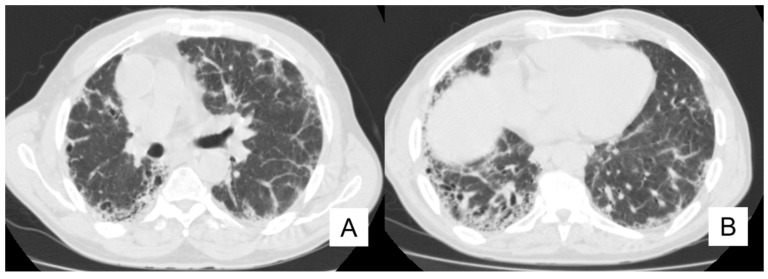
An 80-year-old man with values of SpO_2_, Forced Vital Capacity (FVC) and Forced Expiratory Volume in one second (FEV1) equal to 94%, 57% and 56%, respectively (at first CT scan). A typical IPF pattern was clearly depicted (A,B).
